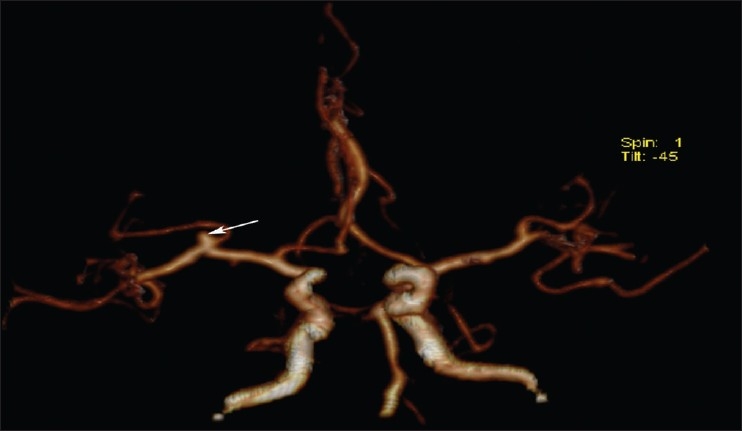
CT angiography showing a right middle cerebral artery aneurysm (white arrow)
